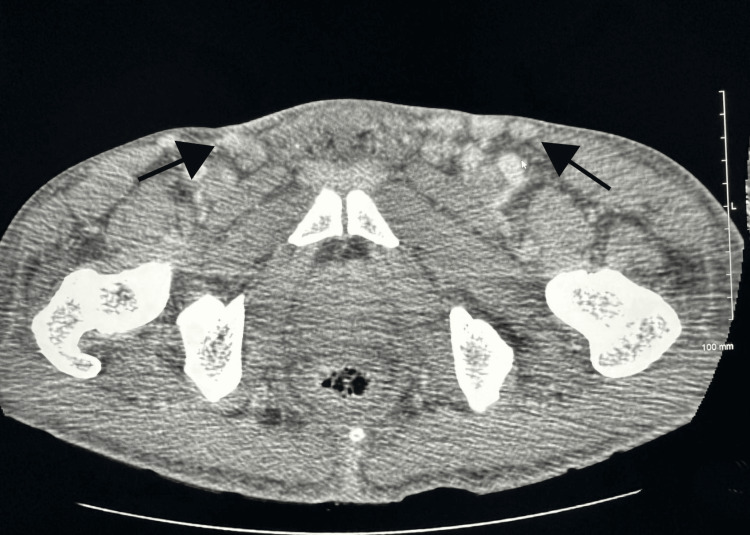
CT of the abdomen and pelvis showing enlarged inguinal lymph nodes (black arrows). CT: computed tomography.
